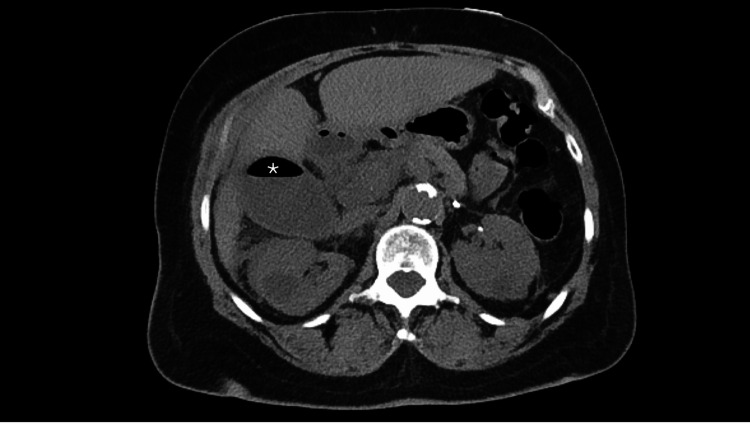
Noncontrasted computed tomography of the abdomen and pelvis demonstrating a large, distended gallbladder with surrounding inflammatory changes and a large collection of gas in within GB (*).
